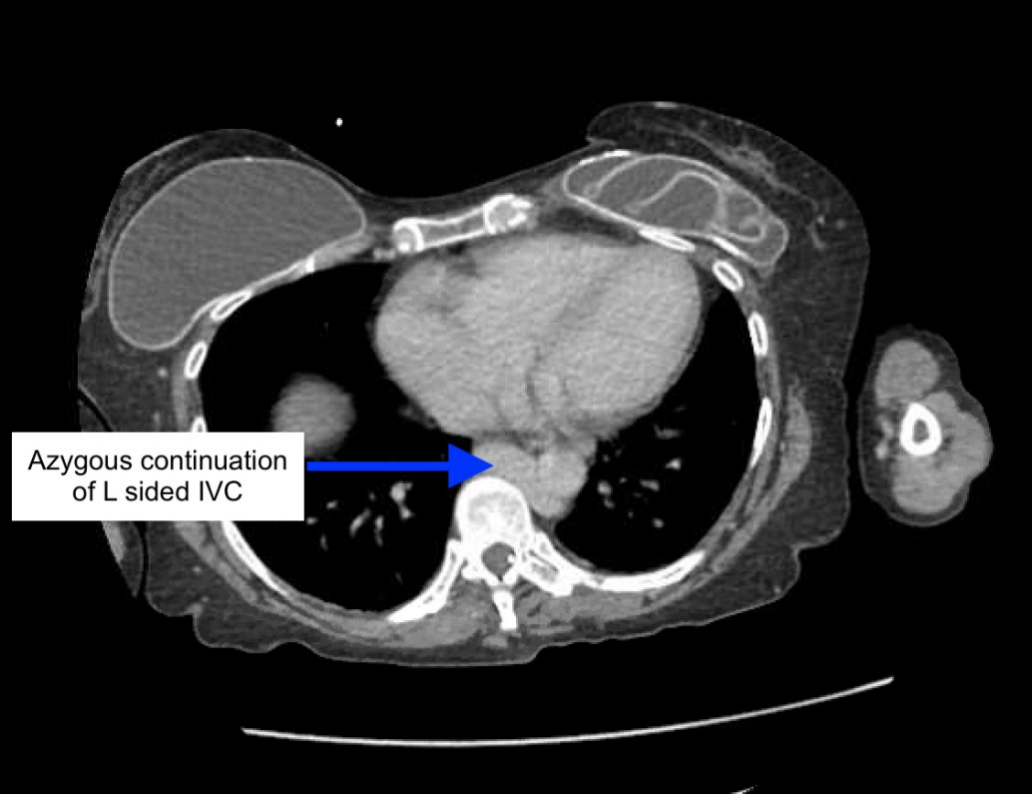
Azygos continuation of L sided IVC (blue arrow). (CT scan abdomen/pelvis w/contrast - axial plane).
